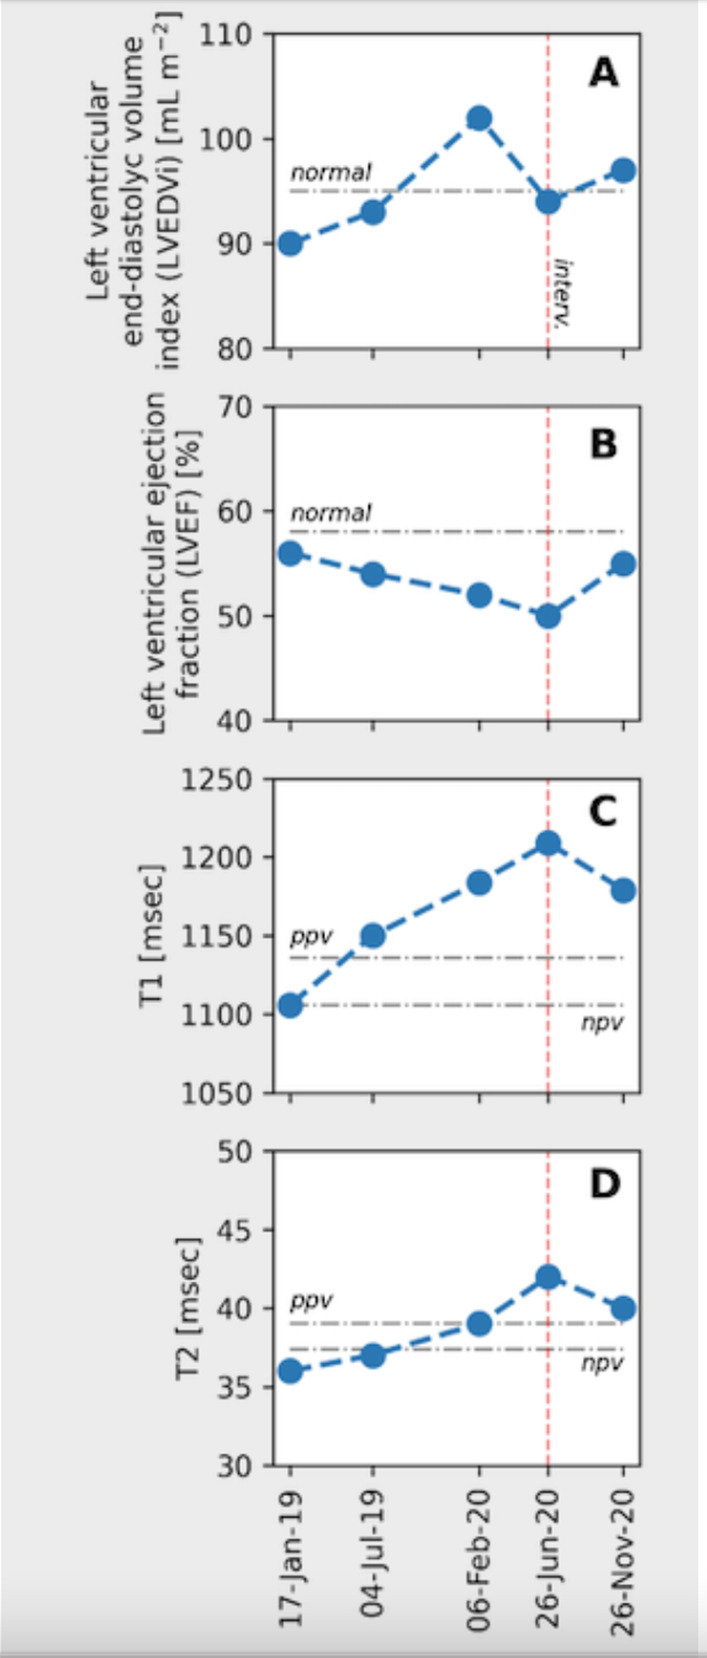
Dynamics of the left ventricular volume, function and cardiac fibrosis, inflammation markers. The horizontal axis shows cardiac MRI scan timepoints, the red vertical line indicating sarcoidosis diagnosis and initiation of the treatment. A Left Ventricular End Diastolic Volume Index increased with time, and normalized after anti-remodeling, anti-inflammatory treatment. B Left ventricular ejection fraction was decreasing and sub-normalized after treatment. C Native T1 value increased drastically and sub-normalized after treatment. D. Native T2 value increased and normalized after treatment. *Ppv-positive predictive value, npv-negative predictive value*
